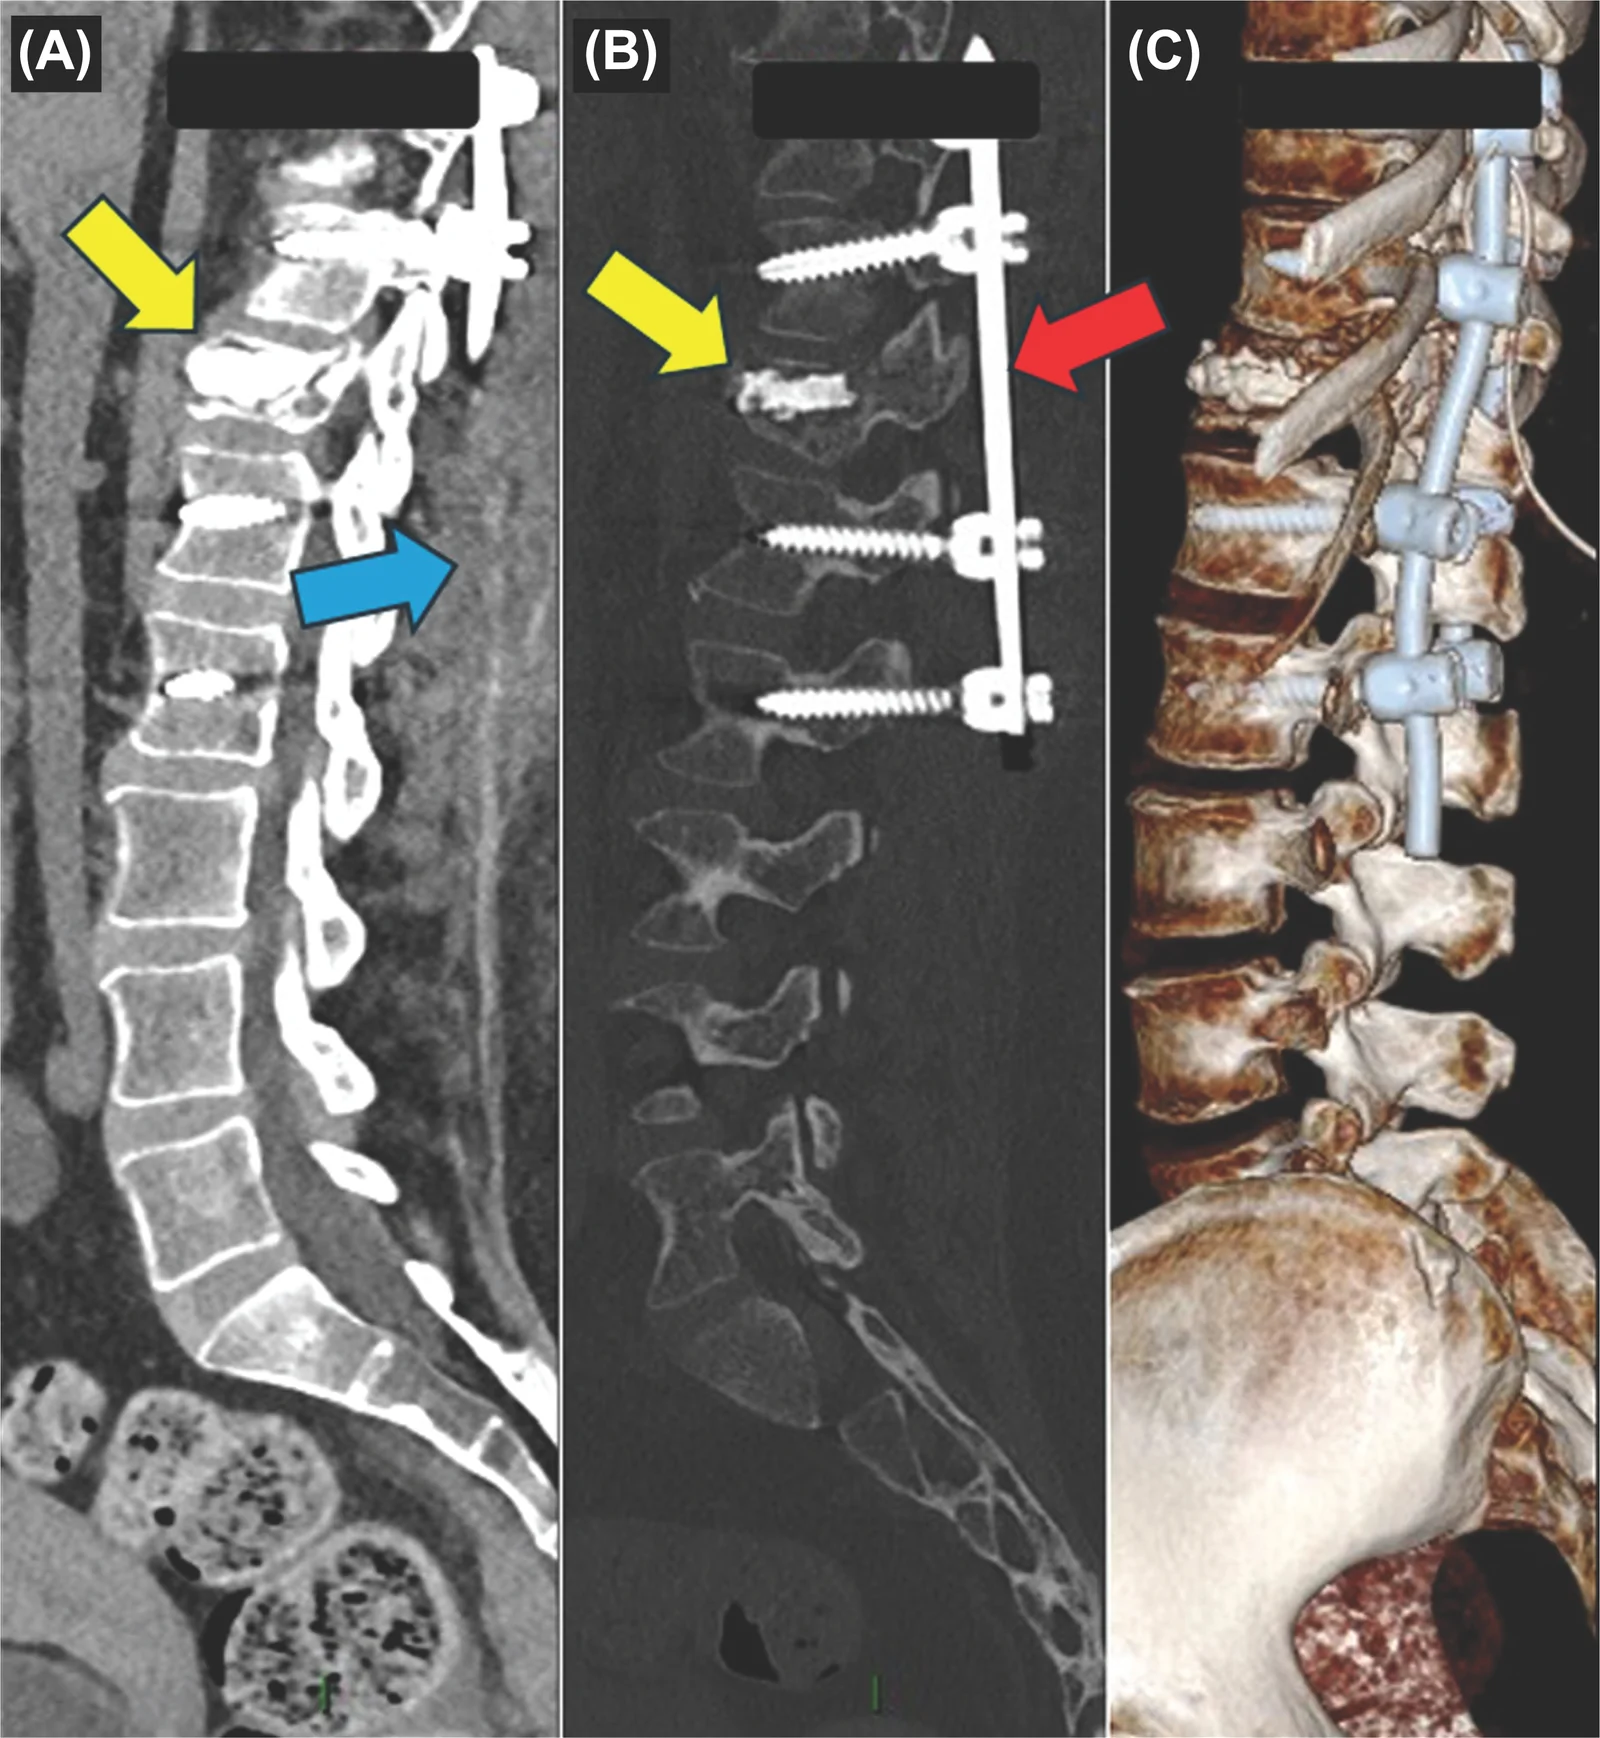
Lumbar spine CT showing sagittal soft-tissue (A), sagittal bone (B), and 3D rendering (C). Post-operative transpedicular screws at T10, T11, L1, and L2 (red arrows). Posterior decompression changes at T11–T12, including laminectomy, hemifacetectomy, drain in place (blue arrows). Cementoplasty at T12 (yellow arrow). Relative loss of lumbar lordosis. Abbreviations: CT, computed tomography; 3D, three-dimensional.
